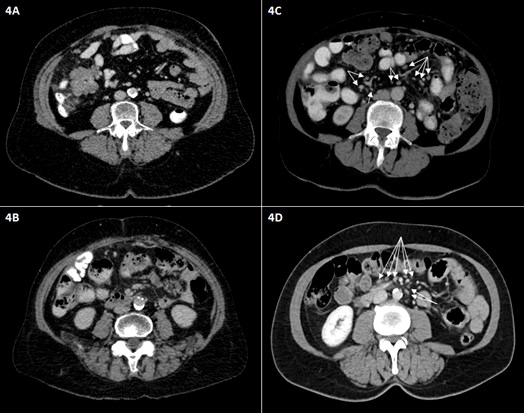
Representative axial intravenous contrast-enhanced CT scans of the middle abdomen. The absence of numerous small mesenteric lymph nodes is shown (A, B); only small vessels in the mesenteric root are visible. (C, D) Other patients show multiple small mesenteric lymph nodes (<1 cm in short dimension) in the mesentery and mesenteric root (arrows).
